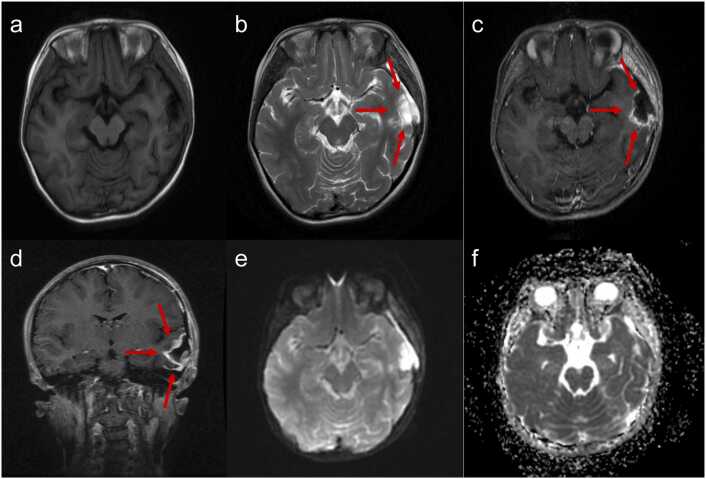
Cranial magnetic resonance imaging 1 month after surgery. An irregular surgical cavity change was observed in the left temporal lobe, measuring 41 × 28 × 52 mm. (a) Axial T1WI: The surgical cavity showed hypointense signal. (b) Axial T2WI: The surgical cavity showed hyperintense signal in the surrounding tissue (indicated by red arrows), with mild displacement of adjacent structures. (c and d) Axial and coronal postcontrast T1 images: The edges of the surgical cavity showed marked ring-like enhancement with an irregular shape. (e and f) Diffusion-weighted imaging and apparent diffusion coefficient maps: The lesion exhibited hyperintense signal in diffusion-weighted imaging and reduced signal in apparent diffusion coefficient maps, respectively, indicating the restricted diffusion.
